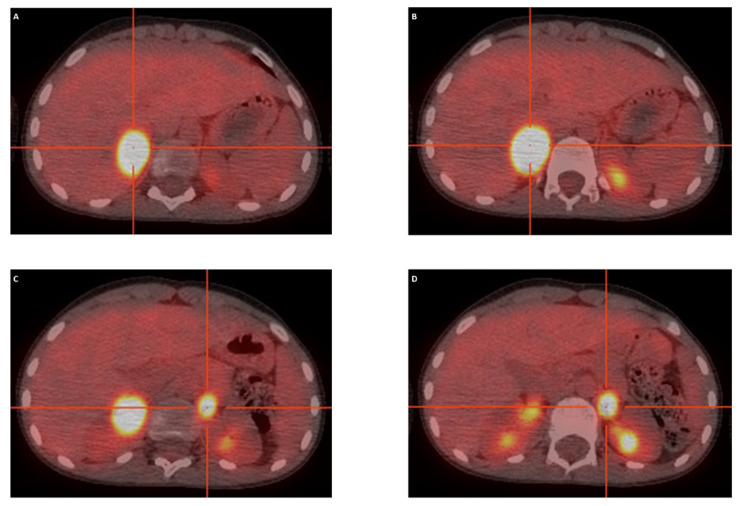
Axial PET/CT images showing bilateral adrenal hypermetabolism (A–D) PET/CT slices confirming intense bilateral adrenal FDG uptake without evidence of distant metastasis. PET/CT: positron emission tomography/computed tomography, FDG: fluorodeoxyglucose
